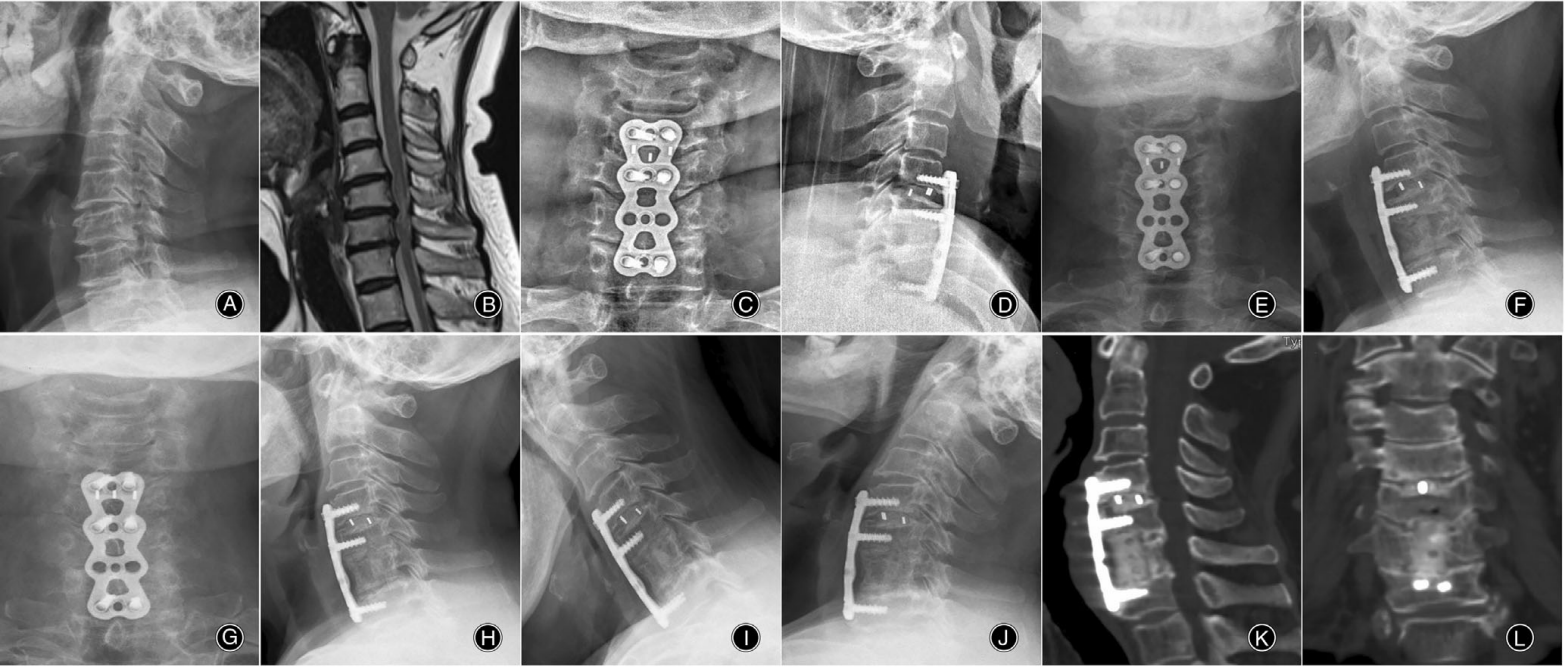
A 54‐year‐old male who underwent corpectomy ACDF hybrid procedures with n‐HA/PA66 cage for multi‐level degenerative cervical myelopathy. Preoperative radiographs (A, B). 1 week X‐rays (C, D), 1 month X‐rays (E, F), 73 months X‐rays (G‐J) and 73 months CT (K, L) postoperative follow‐up radiographs. The X‐rays (G‐J) and three‐dimensional CT scan (K, L) at 73 months after surgery showed bone fusion without internal fixation loosening, breakage, or cage subsidence.
